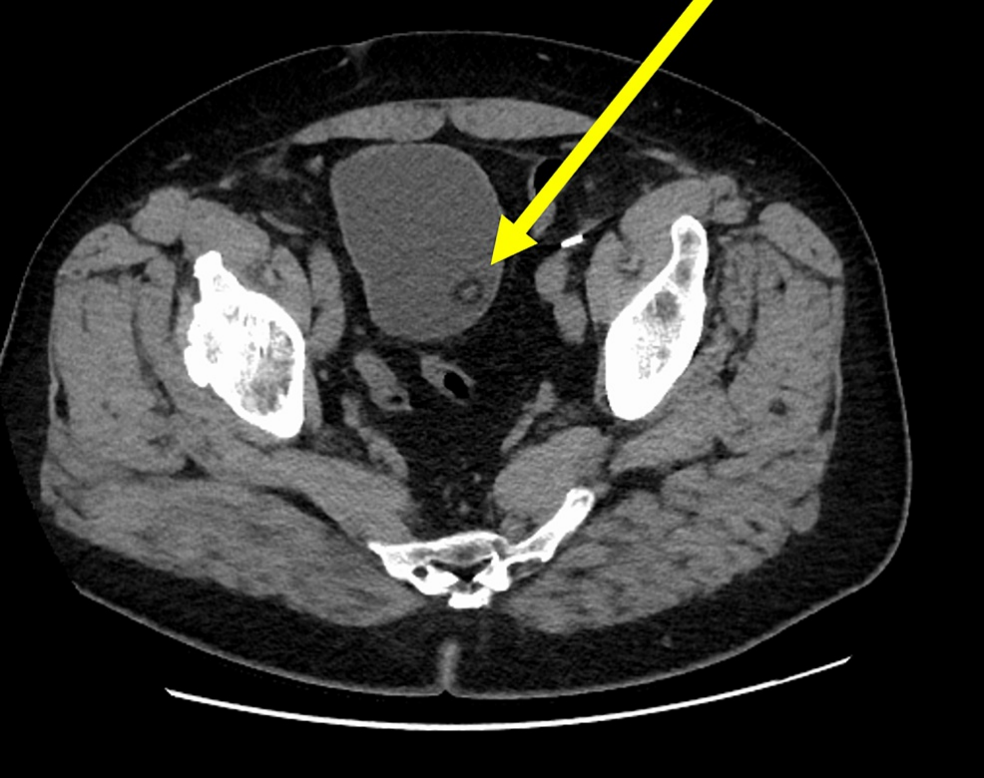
CT Urogram Showing Calcification of Neobladder From Staple Line Erosion
